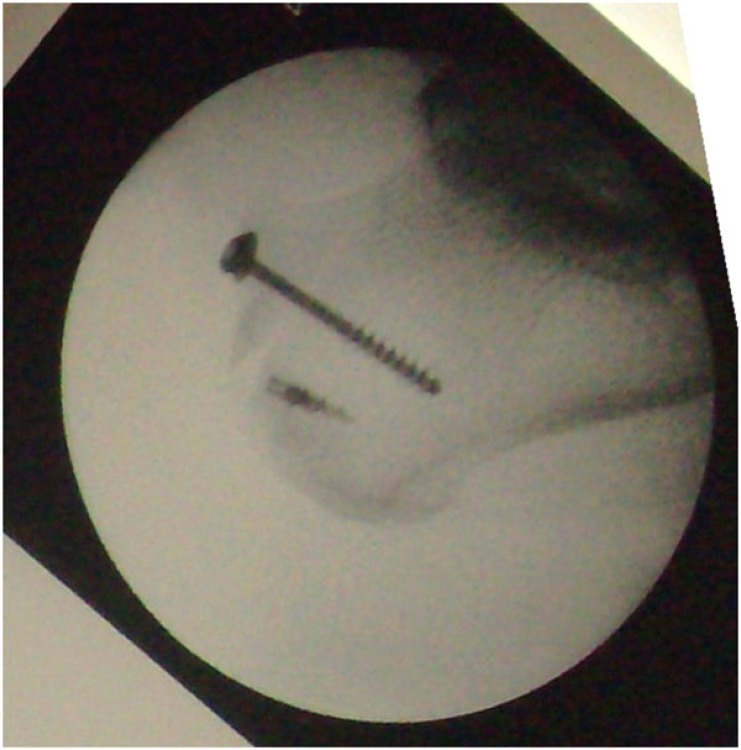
Lateral x-ray after open reduction and internal fixation of avulsed fragment.
